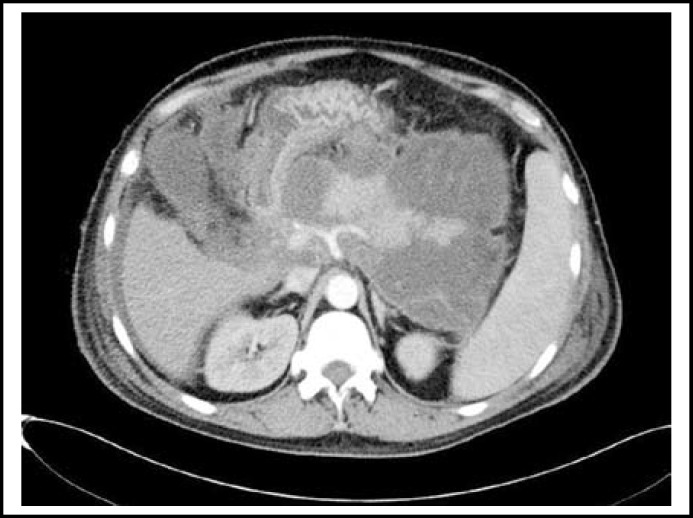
CT confirmed the collections of ﬂuid, and necrotic tissue in the peripancreatic area of Case 1
